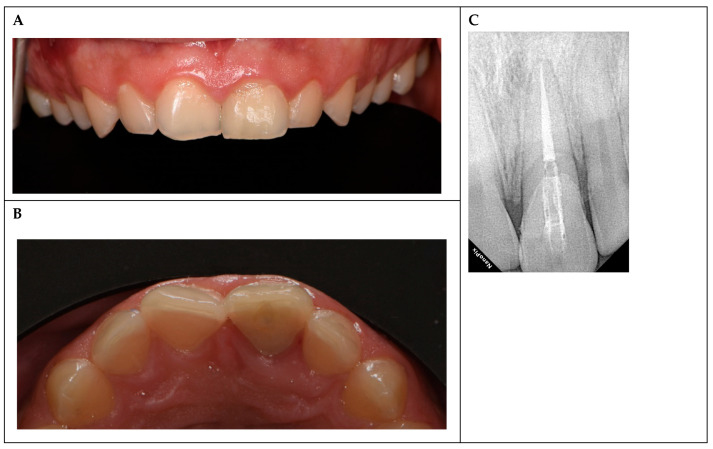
Three-year follow-up. (A) Buccal view showing intact restoration and esthetic outcome. (B) Occlusal view confirming restoration integrity and occlusal harmony. (C) Periapical radiograph at 36 months, indicating no periapical pathology and stable fiber post and restoration.
